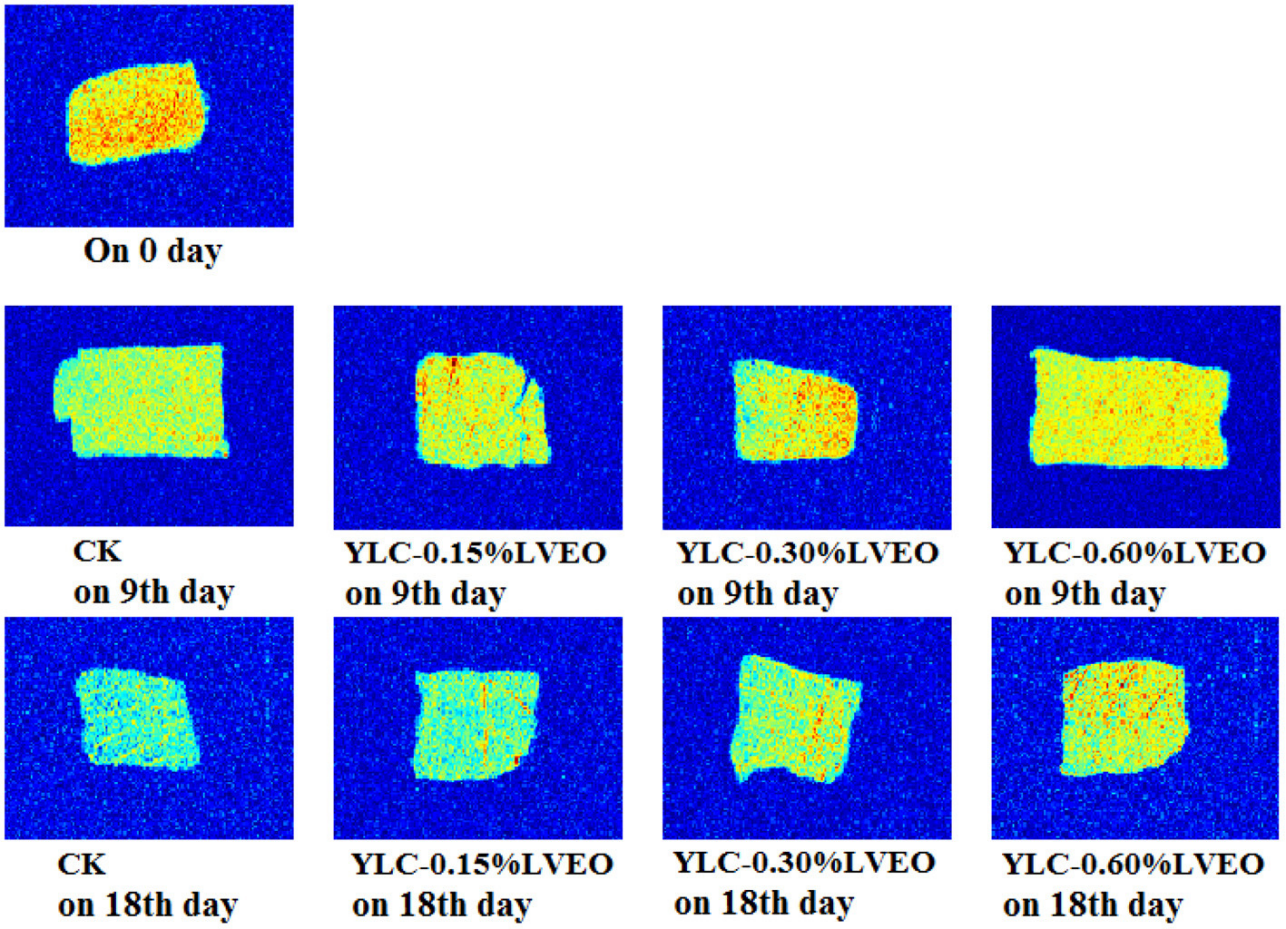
Results of magnetic resonance image (MRI) of large yellow croaker samples under different treatment during refrigerated storage.
